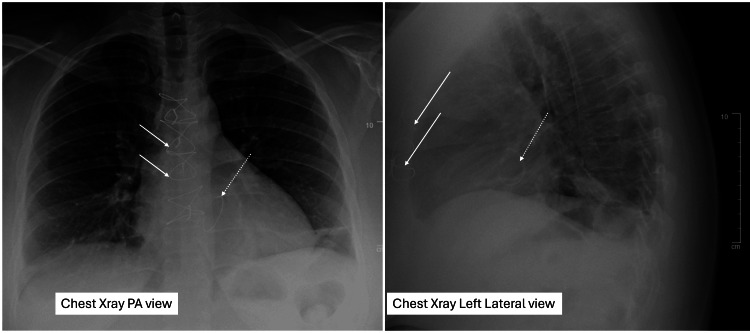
Postoperative chest X-ray showing sternal sutures (white arrows) The mitral annuloplasty ring is also in place (dotted arrows).
